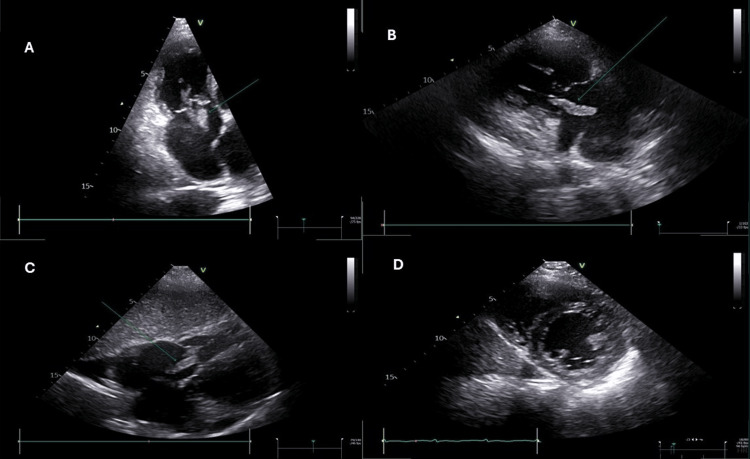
Case 1: The green arrow demonstrating a thrombus passing in and out the right ventricle (RV) across the tricuspid valve in panel A (the apical four-chamber RV dedicated view), panel B (the RV inflow view), and panel C (the subcostal view). Panel D shows the short axis view of papillary muscle level.
